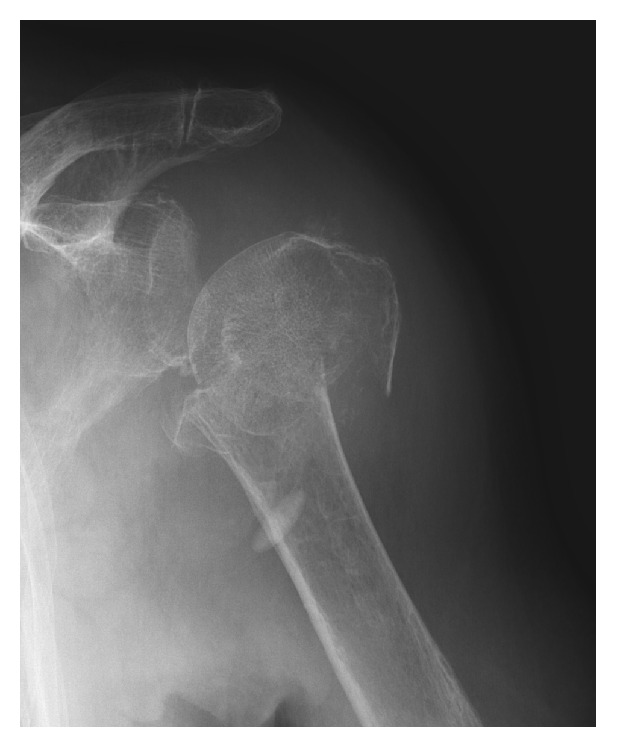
Radiograph of a 3-part proximal humeral fracture of a 96-year-old woman.
